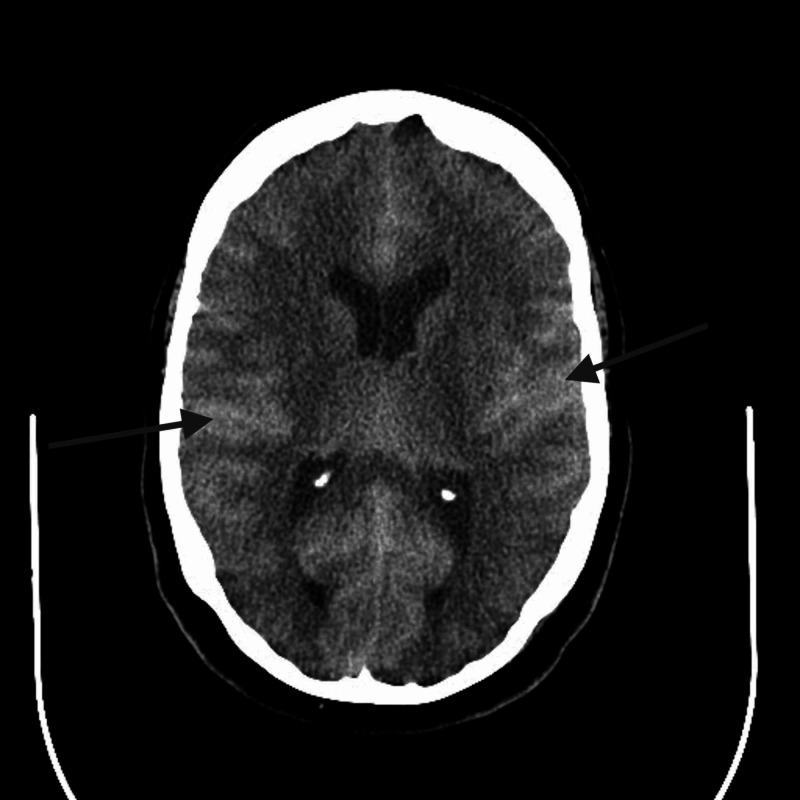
Non-contrast enhanced CT of the brain demonstrating subarachnoid hyperdensity mimicking appearance of a diffuse SAH (arrows point at hyperdensities). CT, computed tomography; SAH, subarachnoid hemorrhage
